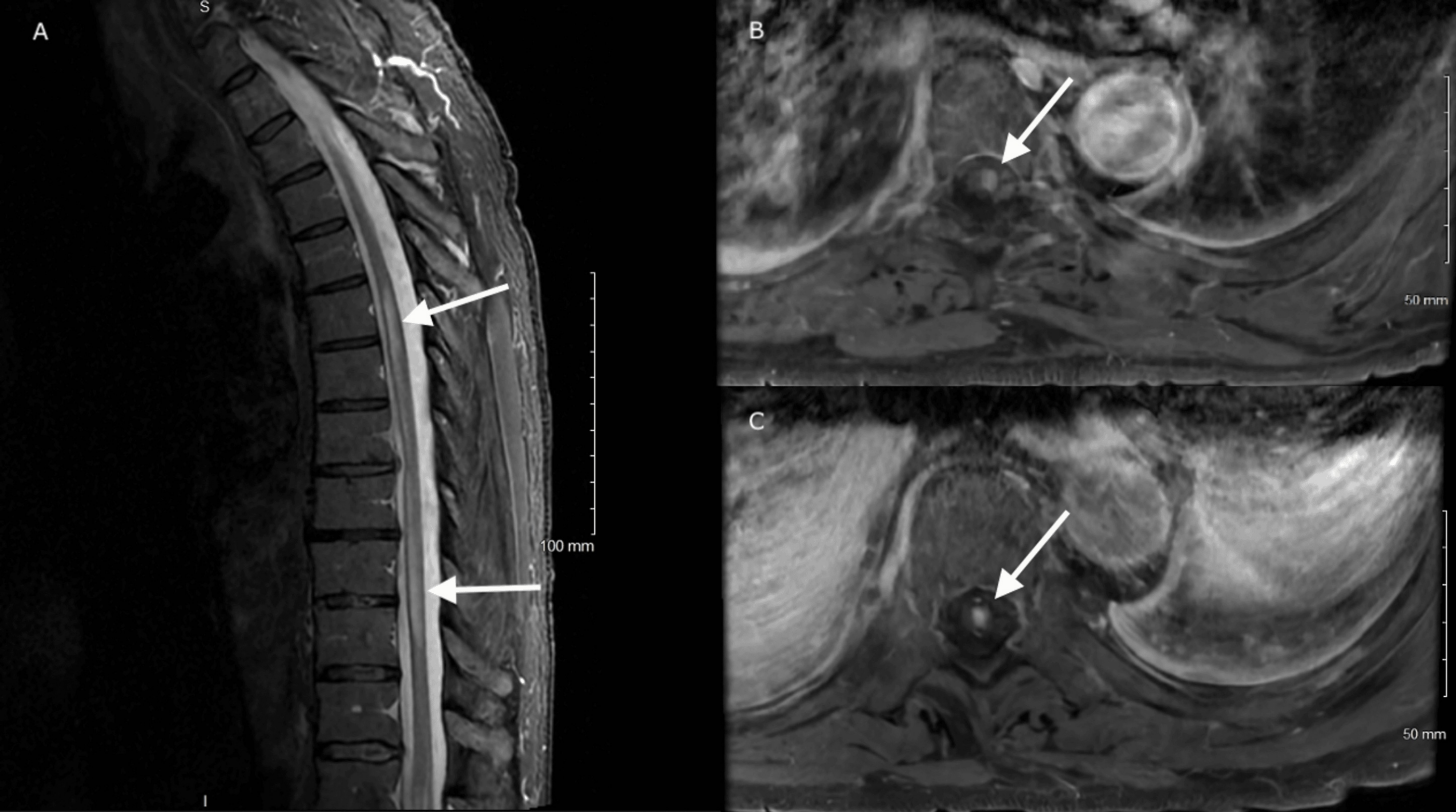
(A) Sagittal and (B and C) axial MRI T2 FLAIR hyperintensities in the thoracic spinal cord. FLAIR: fluid-attenuated inversion recovery
